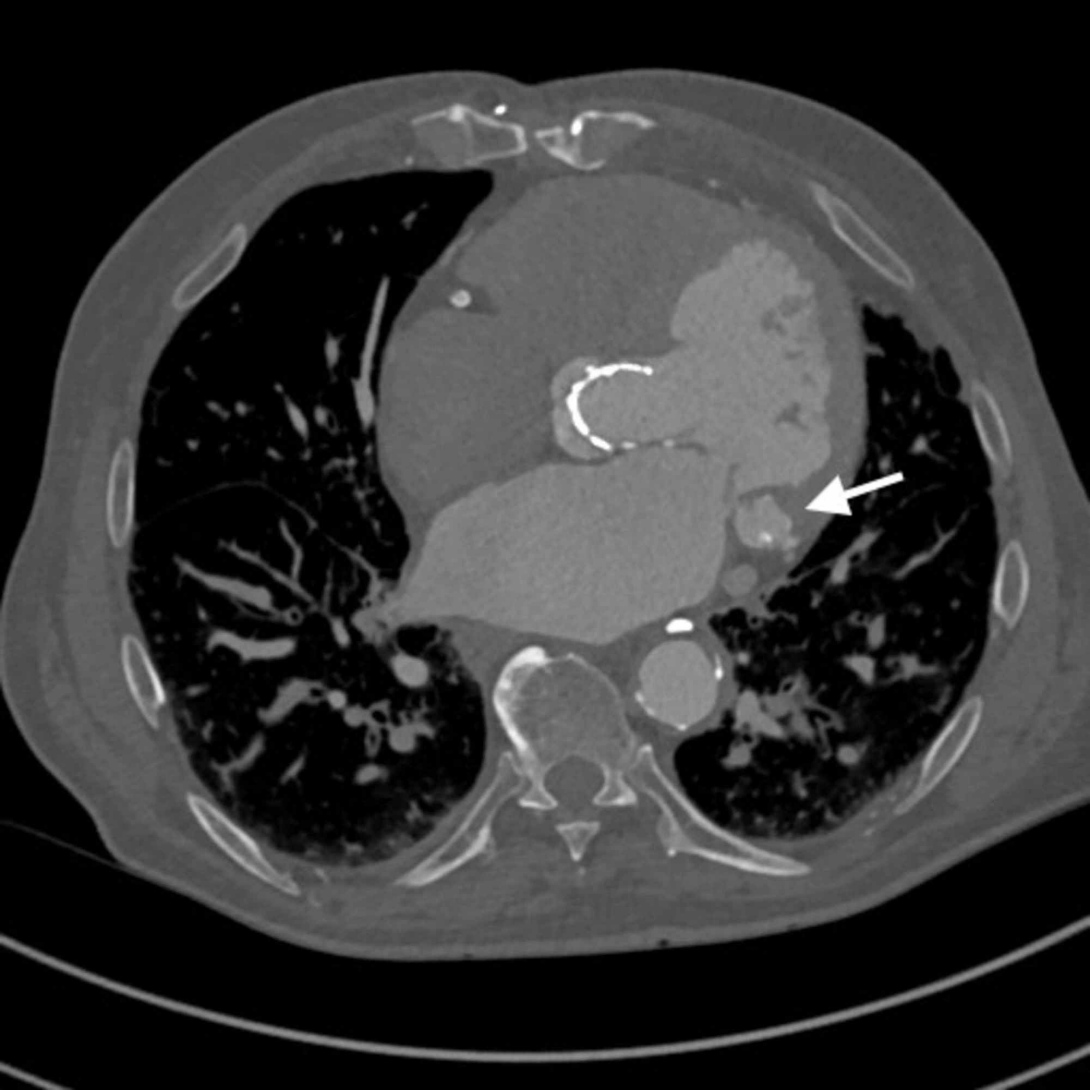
Axial contrast-enhanced coronary CT superiorly to Figure 1. Internal lower density material is seen, raising suspicion for internal necrosis.
